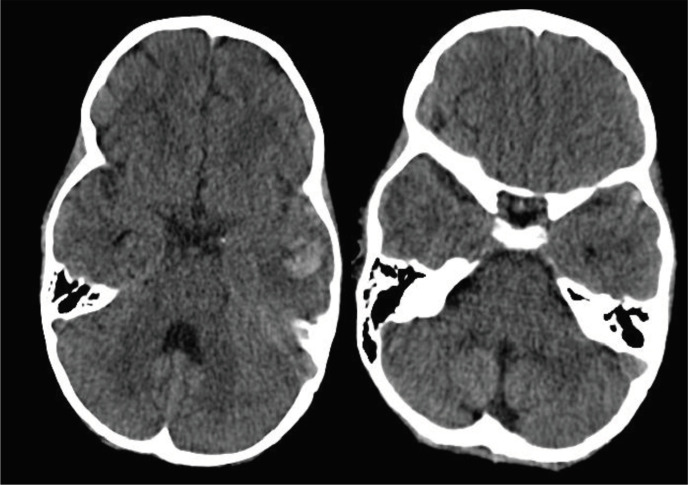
Brain CT: Intraparenchymal hemorrhage in the left temporal lobe with asymmetric increased density in the left lateral sinus
